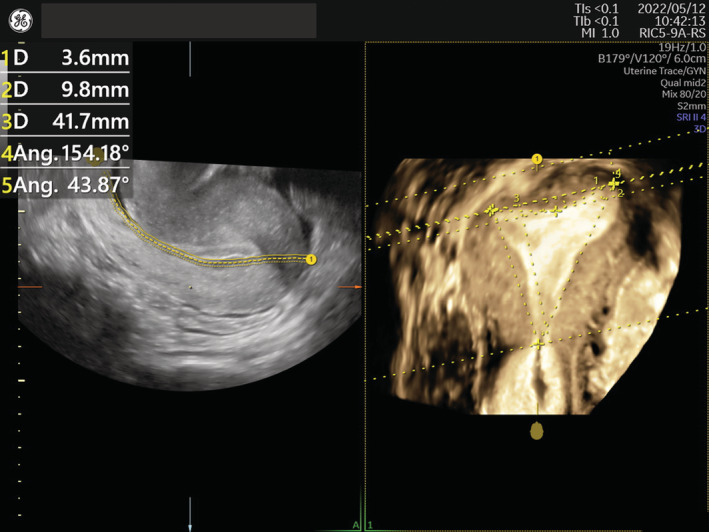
Example image obtained by 3D ultrasound system with volumetric transvaginal probes. Left: the mid‐endometrial line traced on a sagittal plane. Right: measures referring the line connecting the tubal ostium (indicated as yellow crosses) of each side of the uterus on a generated coronal plane.
